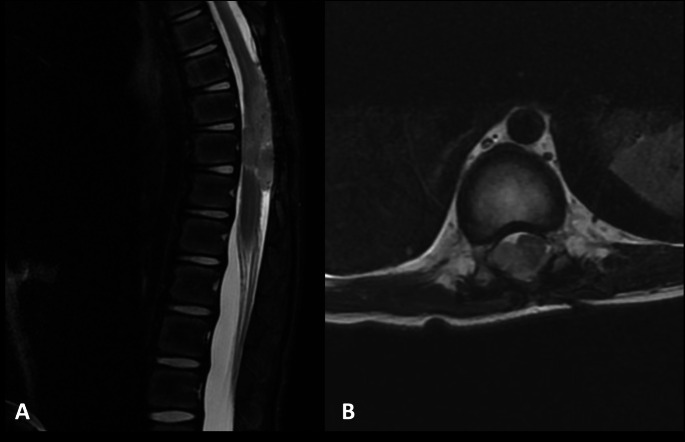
Sagittal (A) and Axial (B) MRI T2 Sequences Displaying Anaplastic Ependymoma in the Thoracic Spine of a 6-months-Old (ID_10). The pronounced compression of the cauda equina is evident. Remarkably, the child exhibited severe spinal pain without accompanying neurological deficits. Surgical intervention involved a laminectomy for tumor resection
